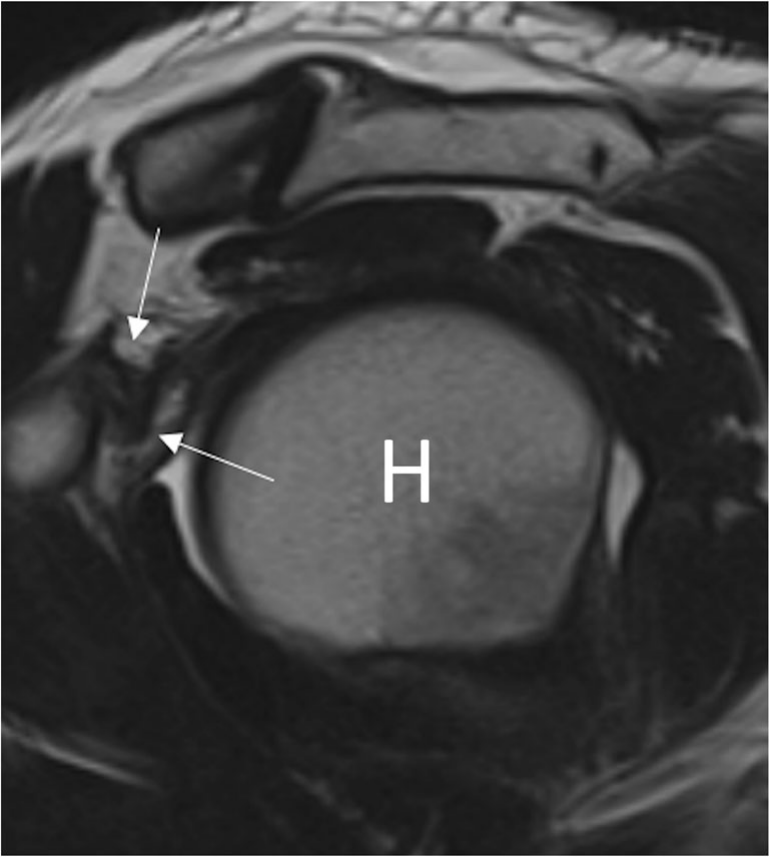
Sagittal oblique T2-weighted image in a patient with adhesive capsulitis. The coracohumeral ligament (*arrows*) is markedly thickened
